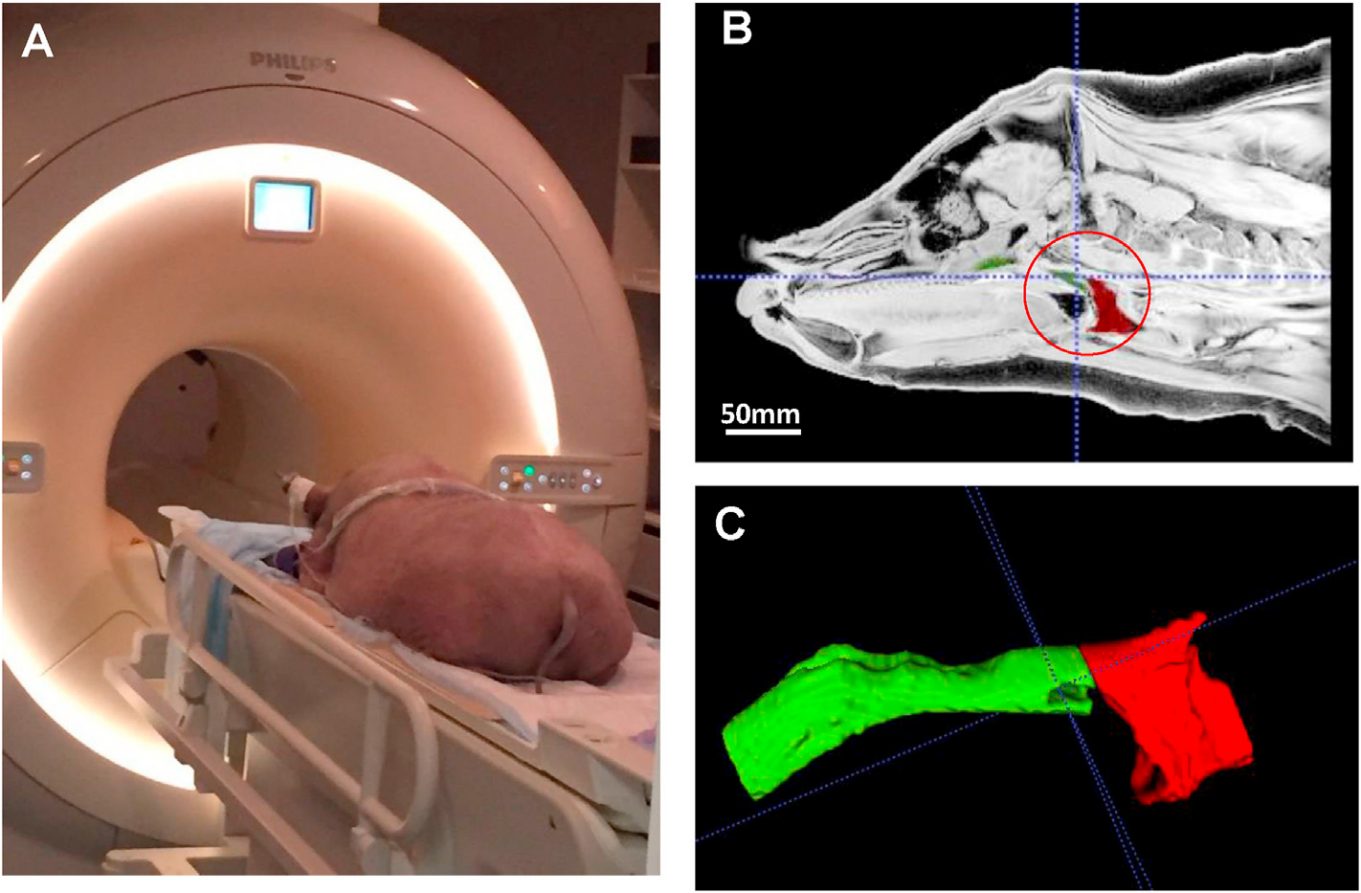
A: MRI imaging in the prone position under sedation. B: Sagittal slice of MRI image. The red circle indicates the region of interest (ROI). C: The segmented 3D model of the nasopharyngeal (green) and the oropharyngeal (red) airway.
